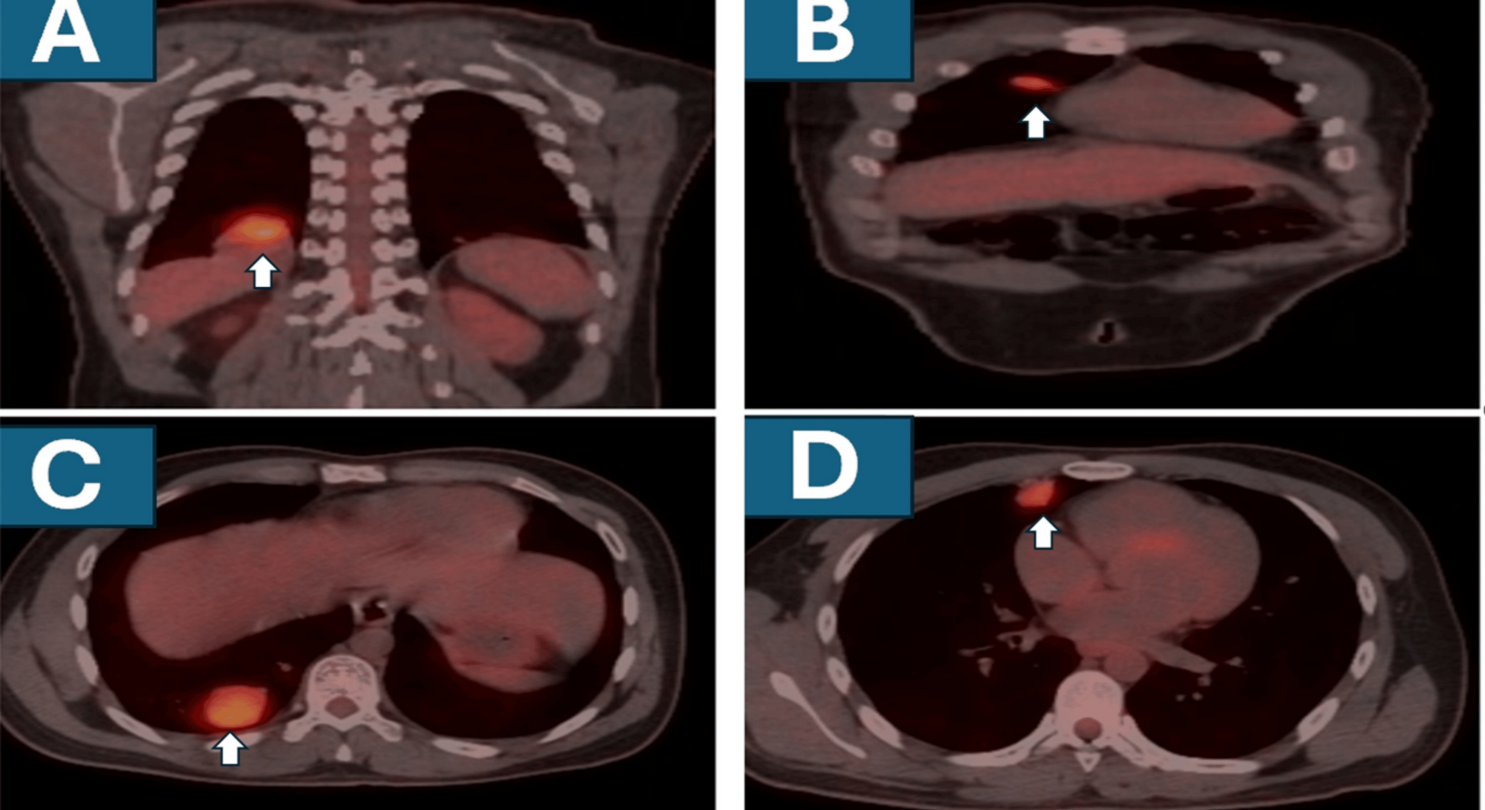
Representative PET/CT images showing progression of the pulmonary metastases A. PET/CT, coronal view showing increased metabolic activity in a pulmonary metastatic lesion on the right above the diaphragm (white arrow). B. Another coronal view showing another pulmonary metastasis with increased metabolic activity in the right lung at a higher level (white arrow). C. PET/CT fused axial image showing a pulmonary lesion with increased metabolic activity posteriorly in the right lung base (white arrow). D. Another axial image showing another pulmonary lesion with increased metabolic activity on the right, posterolateral to the sternum (white arrow).
